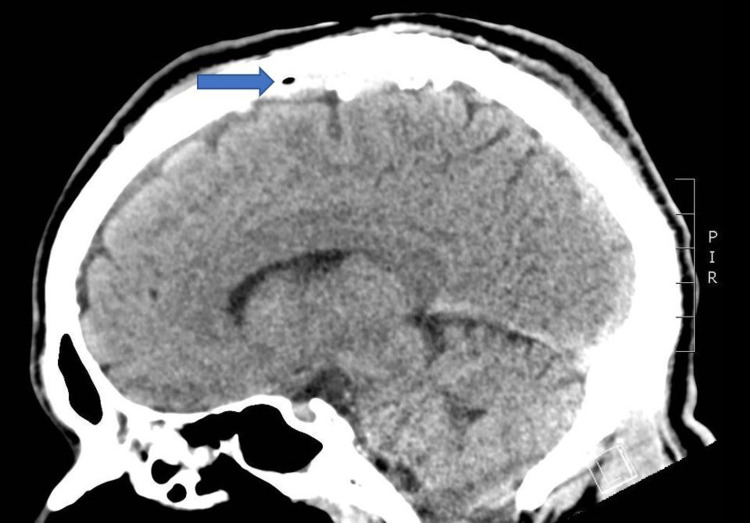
Sagittal slice of a noncontrast CT demonstrating a small focus of air within the sagittal sinus without skull fracture as well as a slight displacement of the superior sagittal sinus from the adjacent skull, concerning for a possible small epidural hematoma measuring 4 mm in thickness without mass effect. CT: computed tomography
